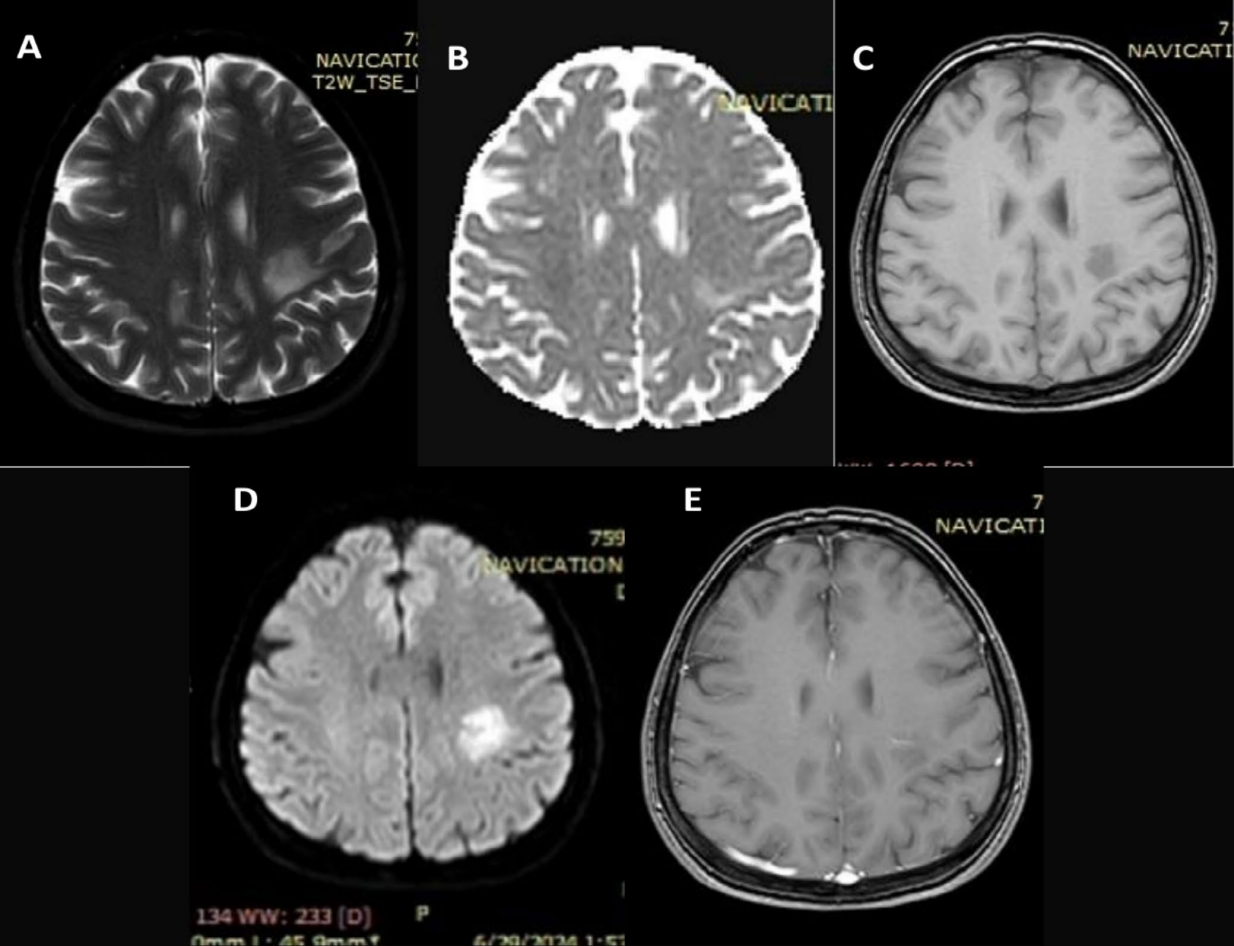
Second brain MRI 4 weeks after infliximab discontinuation and corticosteroid initiation. (A) T2 Axial, (B) ADC, (C) Flair Axial, (D) DWI, (E) T1 Axial Post Contrast. In this magnetic resonance imaging (MRI), regression of the previously enhancing cystic lesion is seen, leaving a small area of T2/FLAIR high SI 20 × 20 mm in size, no diffusion restriction seen nor any enhancement seen apart from the enhanced prominent medullary vein.
